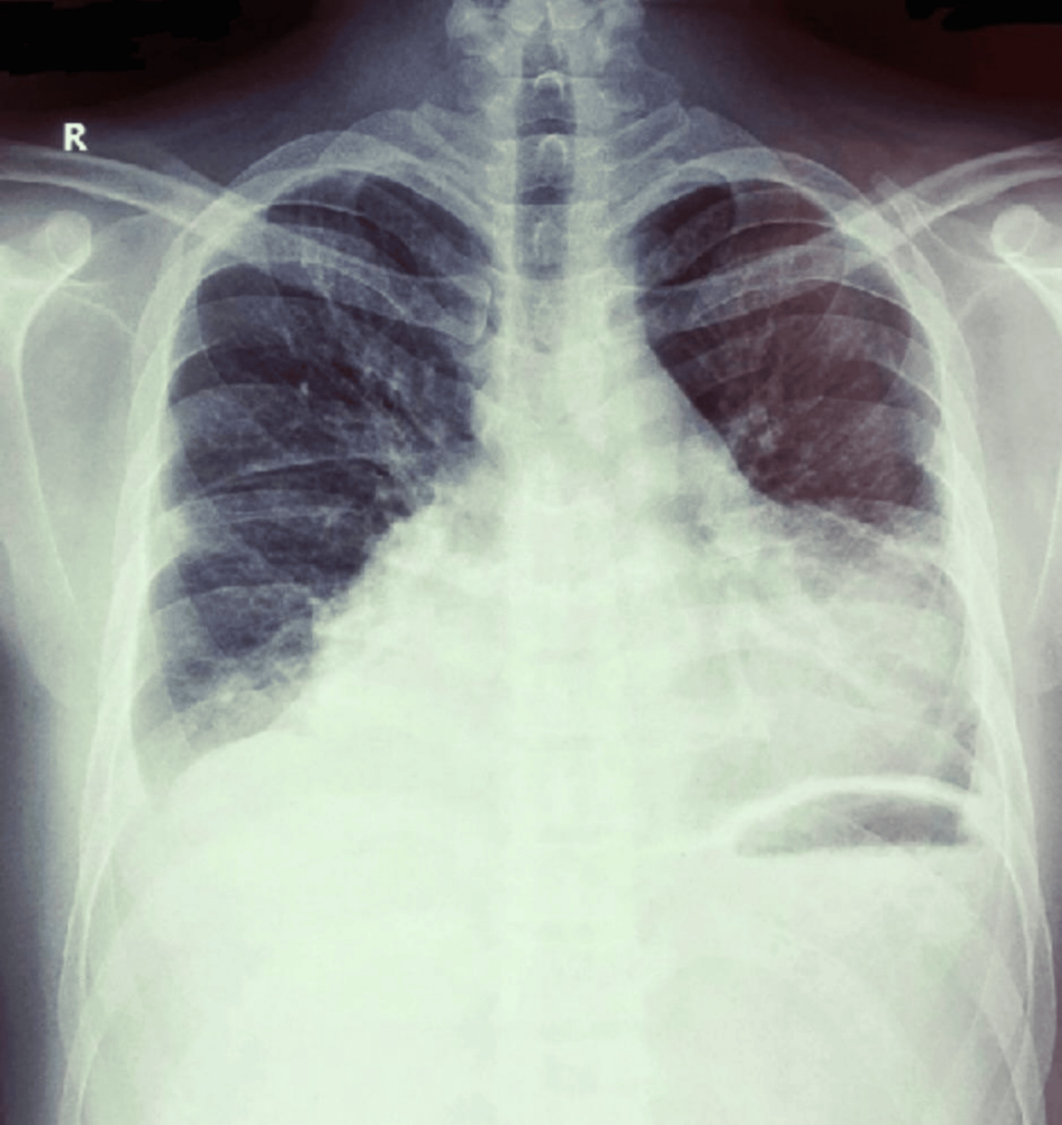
Chest X-ray Chest X-ray shows cardiomegaly with bilateral pleural effusions.
